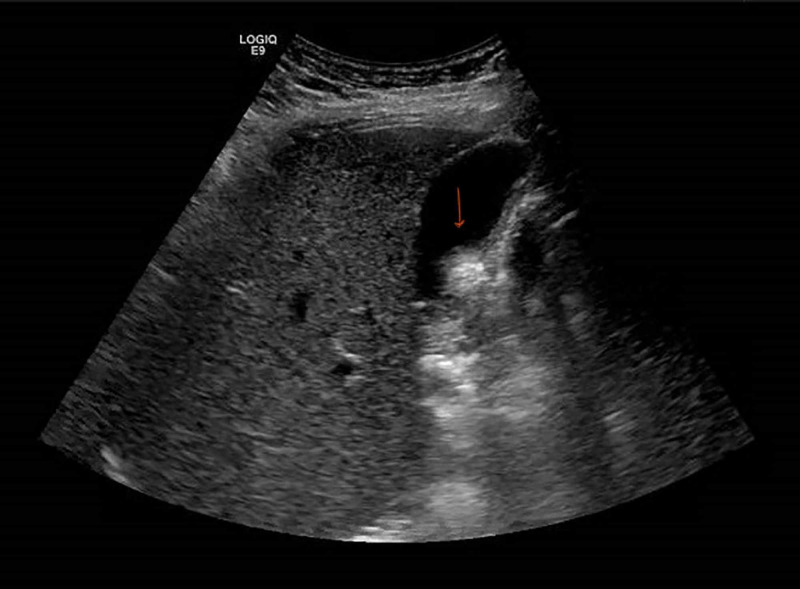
Ultrasound of right upper quadrant of abdomen showing cholelithiasis with normal liver echogenicity
